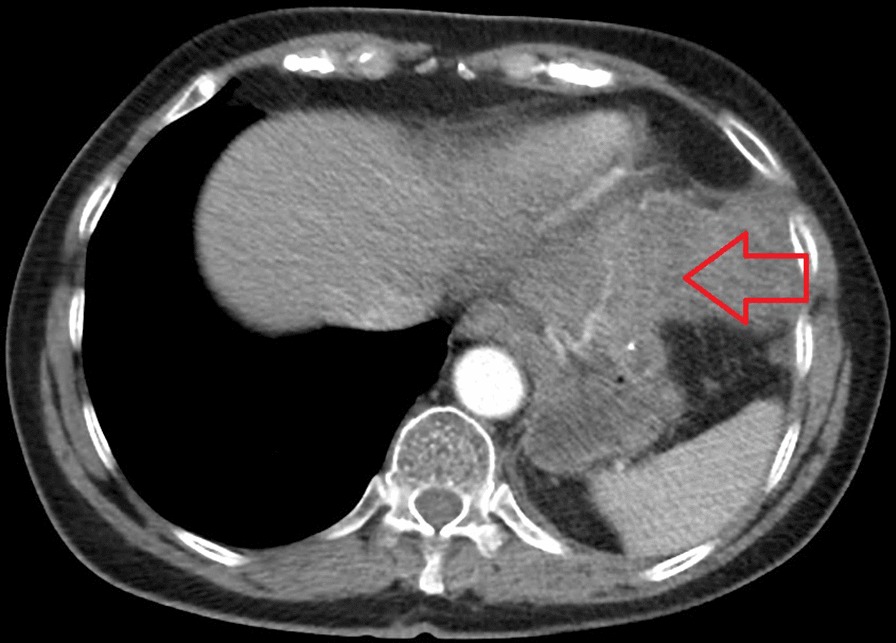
Preoperative cross-sectional CT image of the tumor (red arrow)
